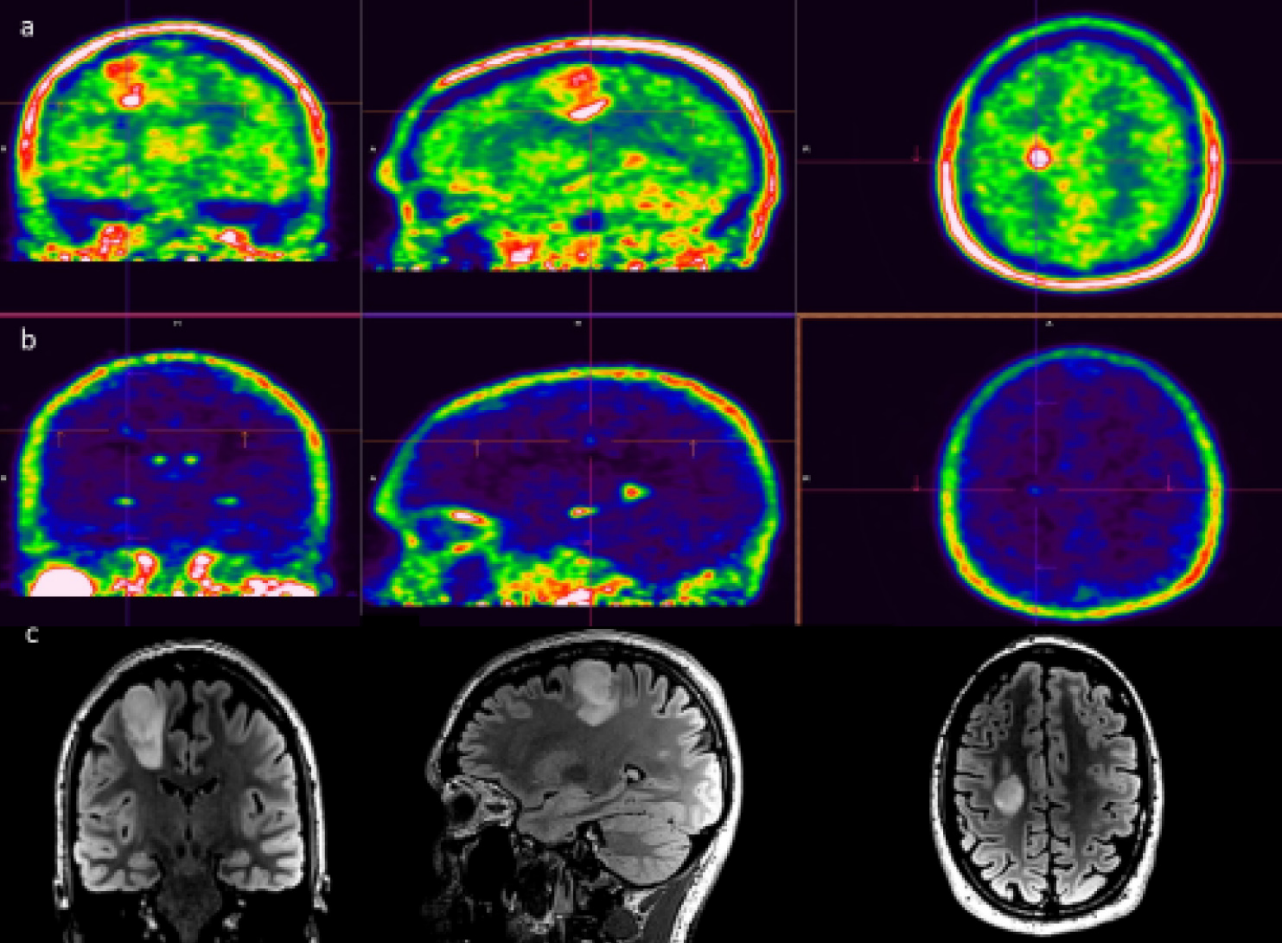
Positron emission tomography-computed tomography (PET-CT) and magnetic resonance imaging (MRI) fluid attenuated inversion recovery (FLAIR) images of a 38-year-old male patient with an isocitrate dehydrogenase 1-mutated 1p/19q-non-codeleted World Health Organization grade II diffuse astrocytoma. O-(2-[^18^F]-fluoroethyl)-L-tyrosine PET was positive (standardized uptake value [SUV] max 2.8) (A) and fluoromethyl-(^18^F)-dimethyl-2-hydroxyethyl-ammonium chloride PET was minimal (SUV max 0.47) (B). MRI FLAIR tumor in the right frontal lobe (C).
